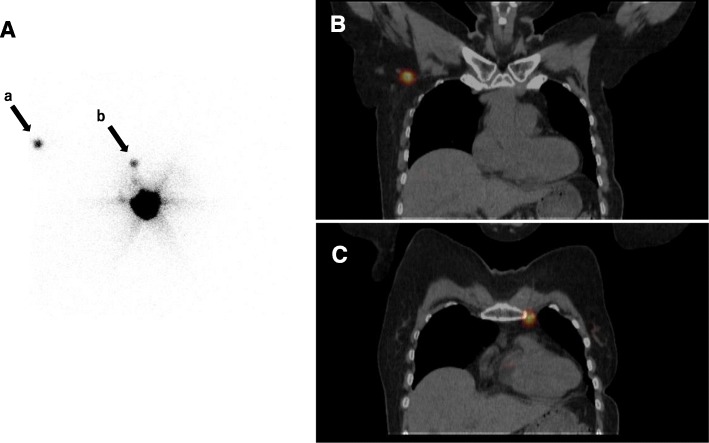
Lymphoscintigraphy and SPECT/CT images of aberrant lymphatic drainages. (A) Lymphatic drainages were visualized at the contralateral axilla (arrow a) and the ipsilateral internal mammary chain (IMC) (arrow b) in a case with left IBTR. SPECT/CT revealed hot spots at the right axillary region (B) and the left IMC (C)
